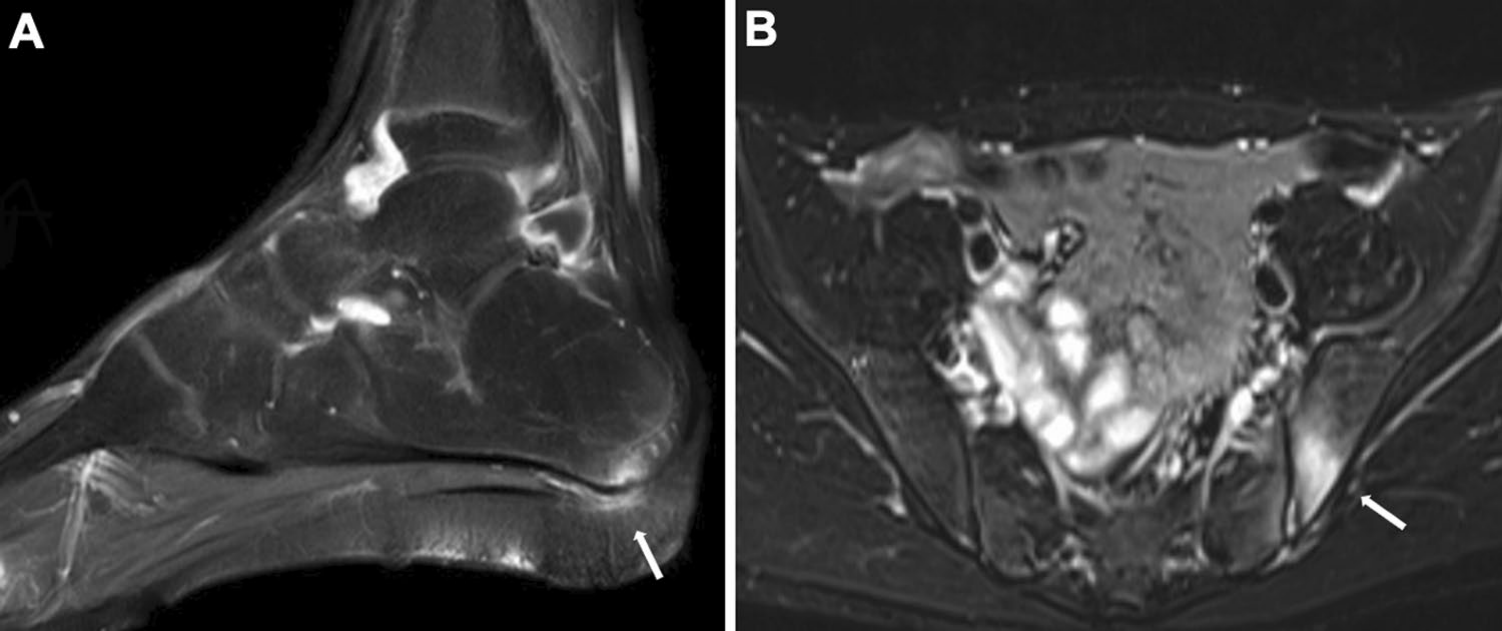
Enthesitis-related arthritis: A–B. A 12-year-old boy with painful left foot and positive HLA B-27. Fat-suppressed-T2-weighted sagittal image showed high signal intensities at the attachment of the plantar fascia to the calcaneus (arrow, A). Three years later, he developed left sacroiliitis that was asymptomatic (arrow, B fat-suppressed-T2-weighted axial image)
